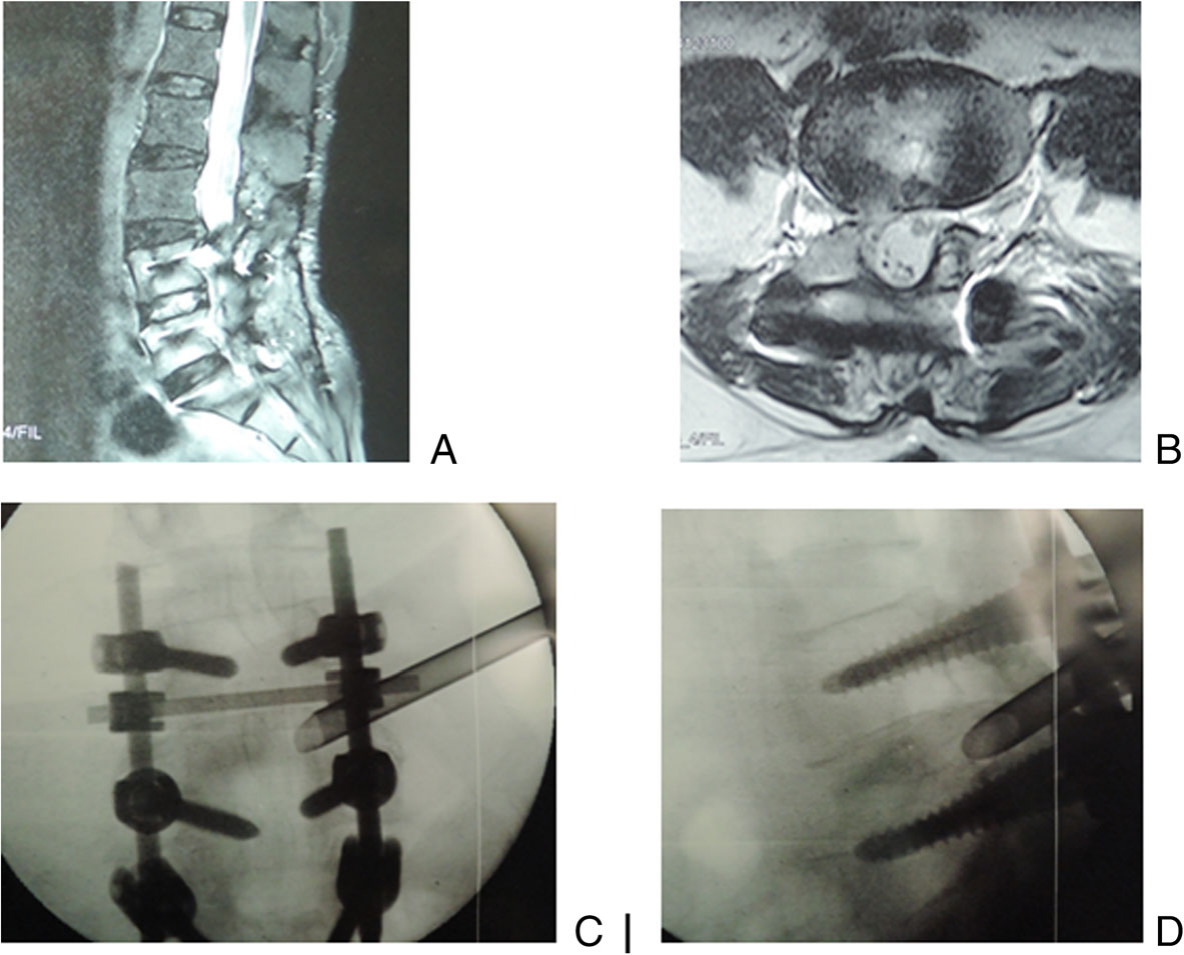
a Sagittal and b axial MR images showed L4/5 recurrent herniation after decompression and fusion in 61-year-old woman. During the procedure of PTES, c posteoanterior and d lateral C-arm image confirmed that a 7.5-mm working cannula was advanced directly to the protruding fragment
